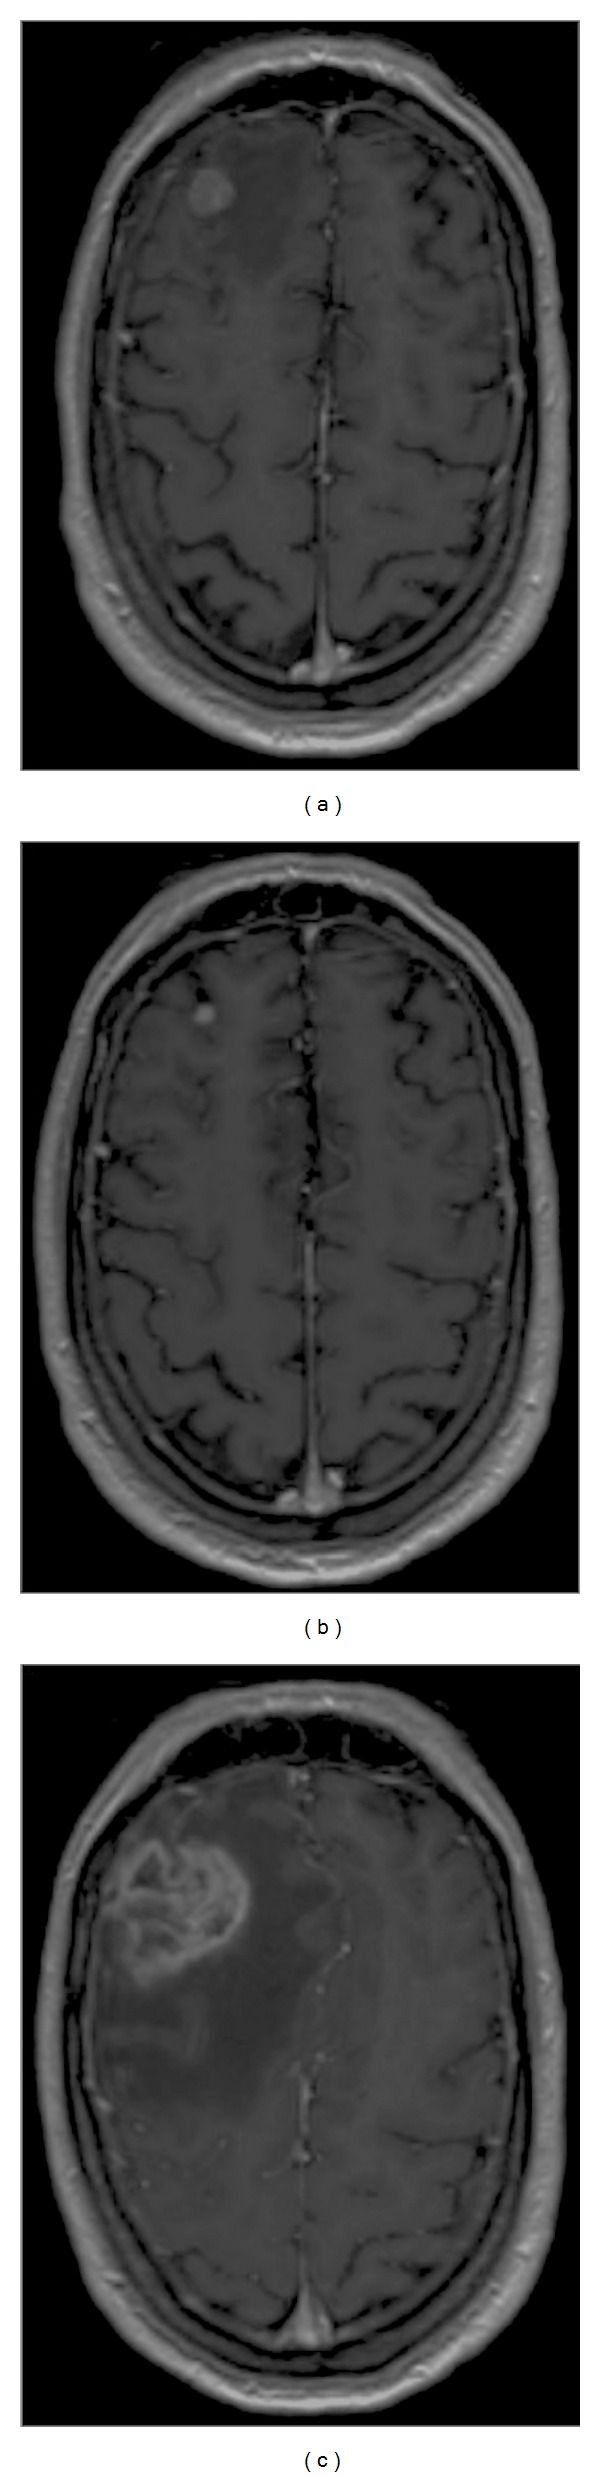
Gadolinium-enhanced T1-weighted MRI images obtained (a) at diagnosis, (b) 6 months after SRT, and (c) at occurrence of symptoms 9 months after SRT.
